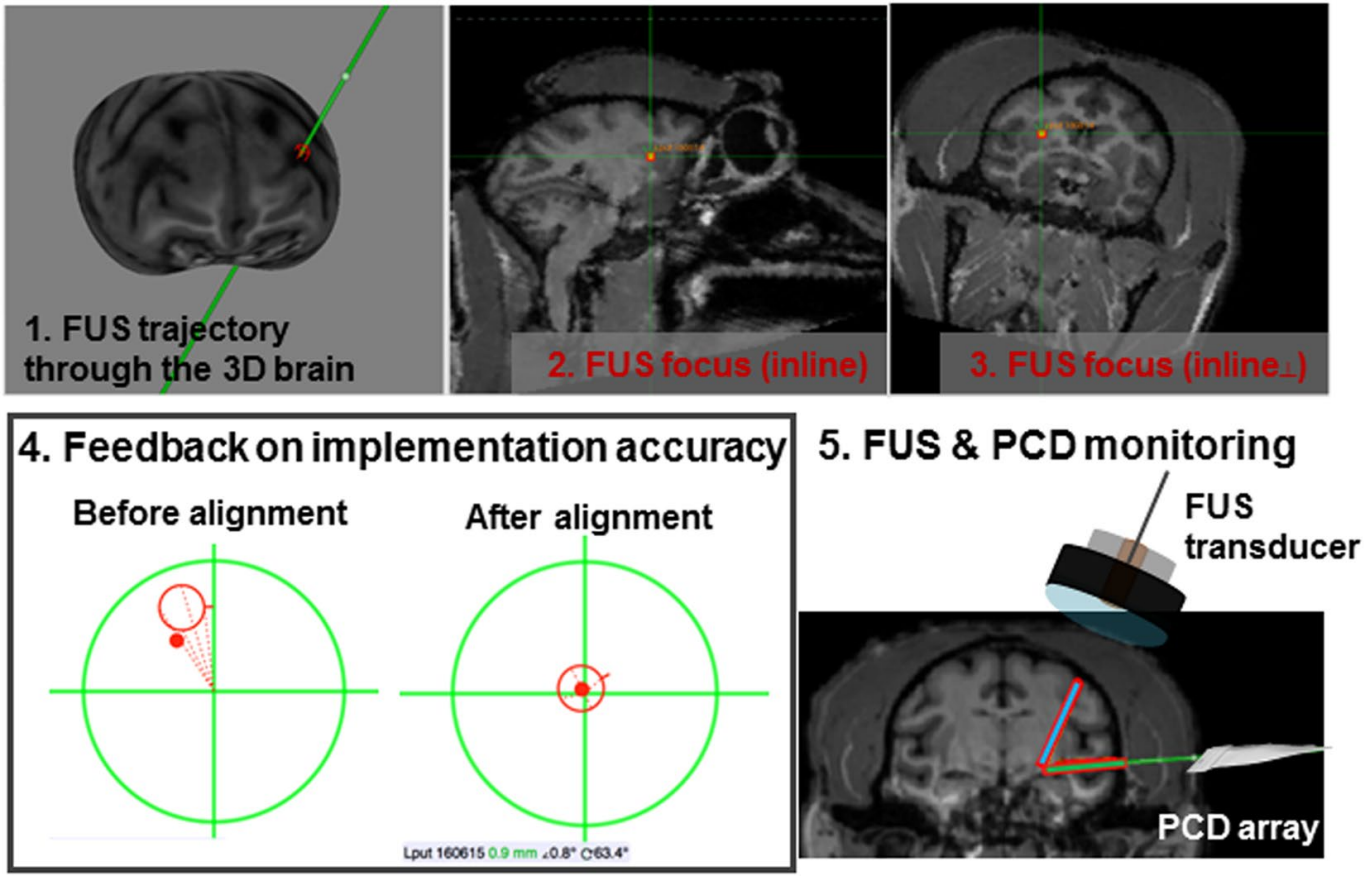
Screenshots of the neuronavigation-guided FUS session. Online session of the FUS procedure showing the FUS trajectory in the reconstructed 3D brain (1) targeted the putamen in two orthogonal MRI slices in line with the FUS transducer, where the vertical arrows represent the FUS trajectory pointing at the focus (2–3). (4) The implementation accuracy of the FUS transducer to the preplanning was displayed as a feedback for the distance (visualized as the distance between the red dot and the center of the larger circle) and the angle deviation (visualized as the distance between the red dot and the center of the smaller circle) during the guiding process. (5) The monitoring transducer for acoustic mapping was aligned to the FUS focus before sonication with neuronavigation guidance.
